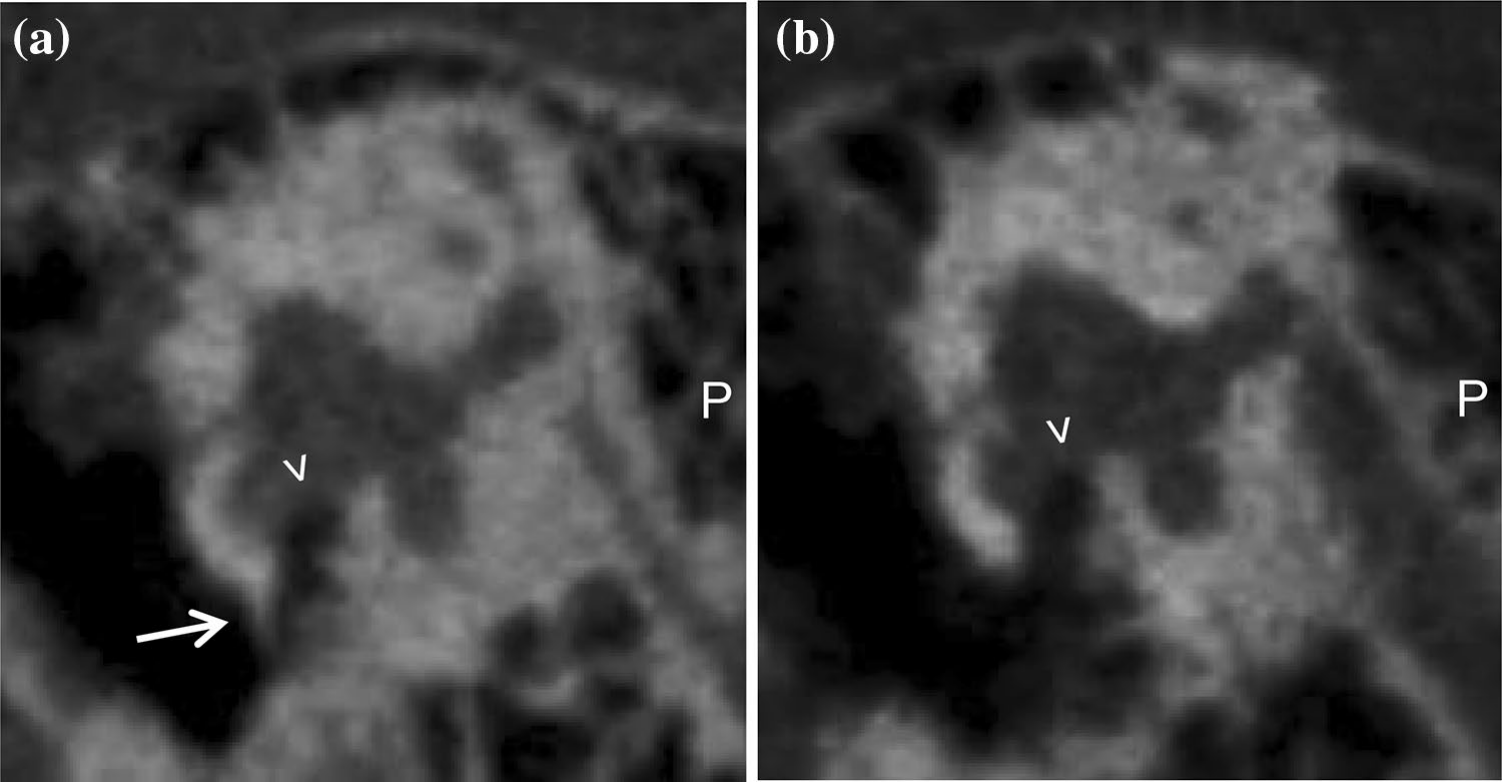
Sagittal slices of temporal bone CBCT. The RWN ( >) can be clearly identified on the right (a) and on the left (b) patient side. On the right side there is an additional bony overhang ( →), while the left side seems open
